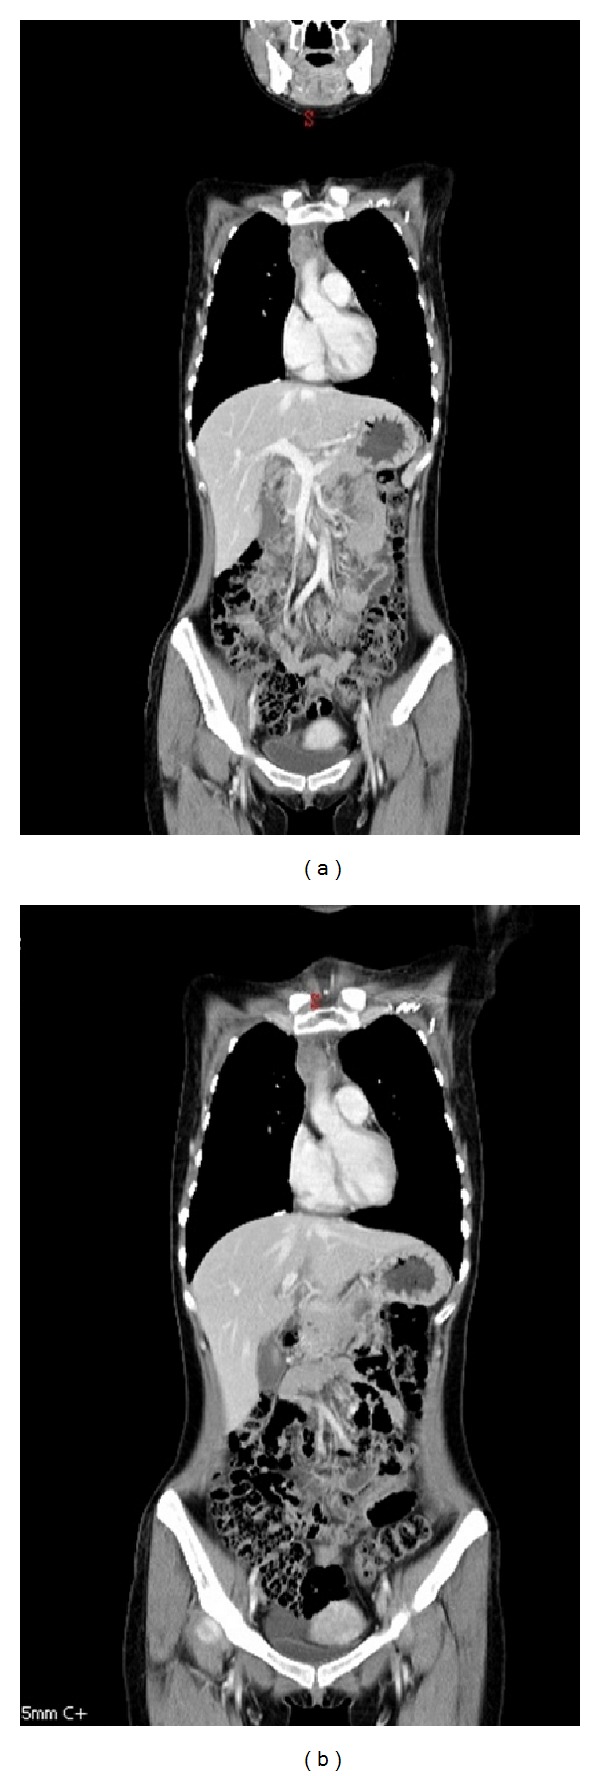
Mediastinal tumor refractory to salvage radiotherapy. (a) CT shows relapsed superior mediastinal tumor before radiotherapy. (b) CT reveals progression of the superior mediastinal tumor 2 months after completion of radiotherapy.
